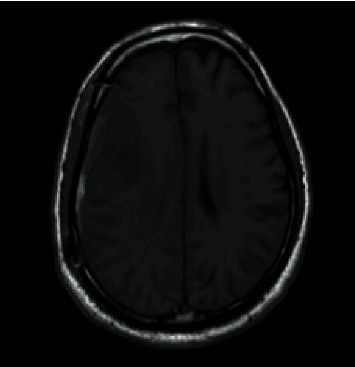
Axial T1-weighted MRI brain showing a subdural collection with mixed density along the right convexity.
